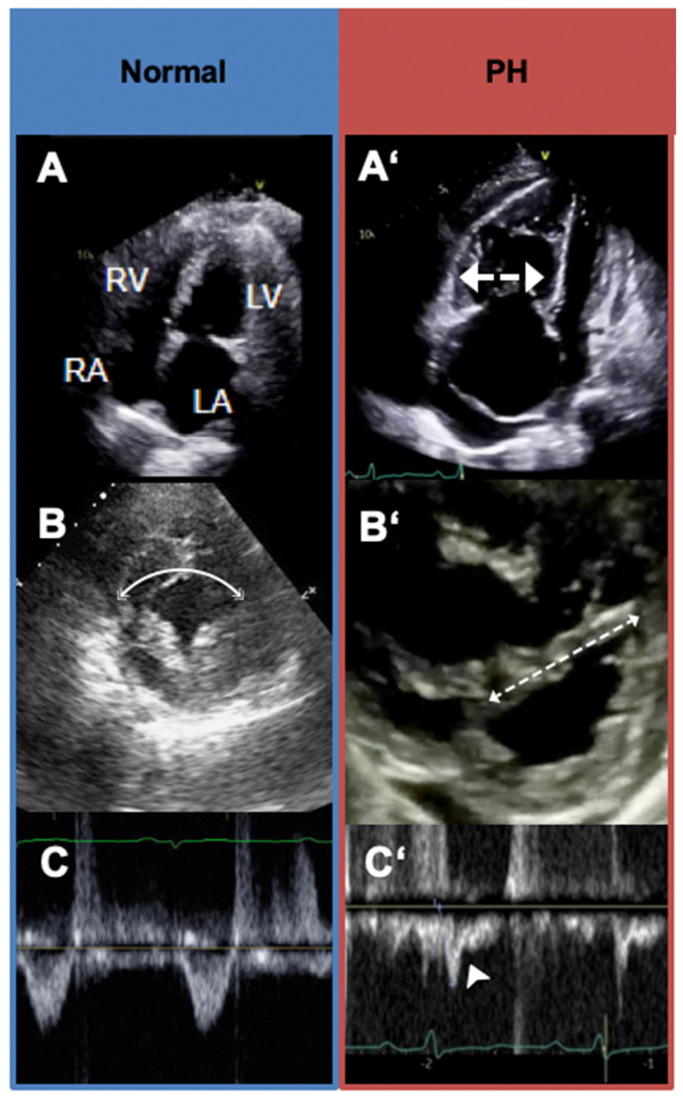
Transthoracic echocardiogram findings of precapillary pulmonary hypertension. In normal physiology, the right ventricle (RV) is approximately 2/3 of the size of the left ventricle (LV); the LV forms the apex of the heart, and the RA is similar in size to the left atrium (A). The interventricular septum (IVS) is round ((B), line), and the right ventricular outflow tract pulse wave Doppler profile (RVOT PWD) is parabolic (C). In pulmonary arterial hypertension, the RV is enlarged and apex-forming ((A’), arrow), with right atrial enlargement (A’). The IVS can flatten in systole (“pressure“ overload or high resistance) (B’). The RVOT PWD is notched in appearance ((C’), arrowhead). RA = right atrium, RV = right ventricle, LA = left atrium, LV = left ventricle.
